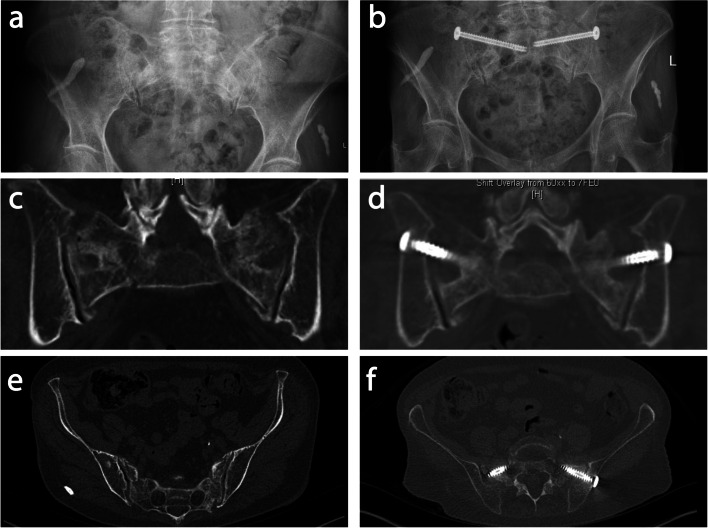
X-ray and CT pictures of one patient showing a bilateral pelvic injury before and after the surgery. a X-ray picture, shows a rather inconspicuous finding, before and (b) after the surgery. c Additional CT-diagnostics (coronar view) clearly shows an injured pelvis (FFP 4b classification) of a patient before and (d) after the surgery. e CT picture of the same patient before and (f) after the surgery in axial view
